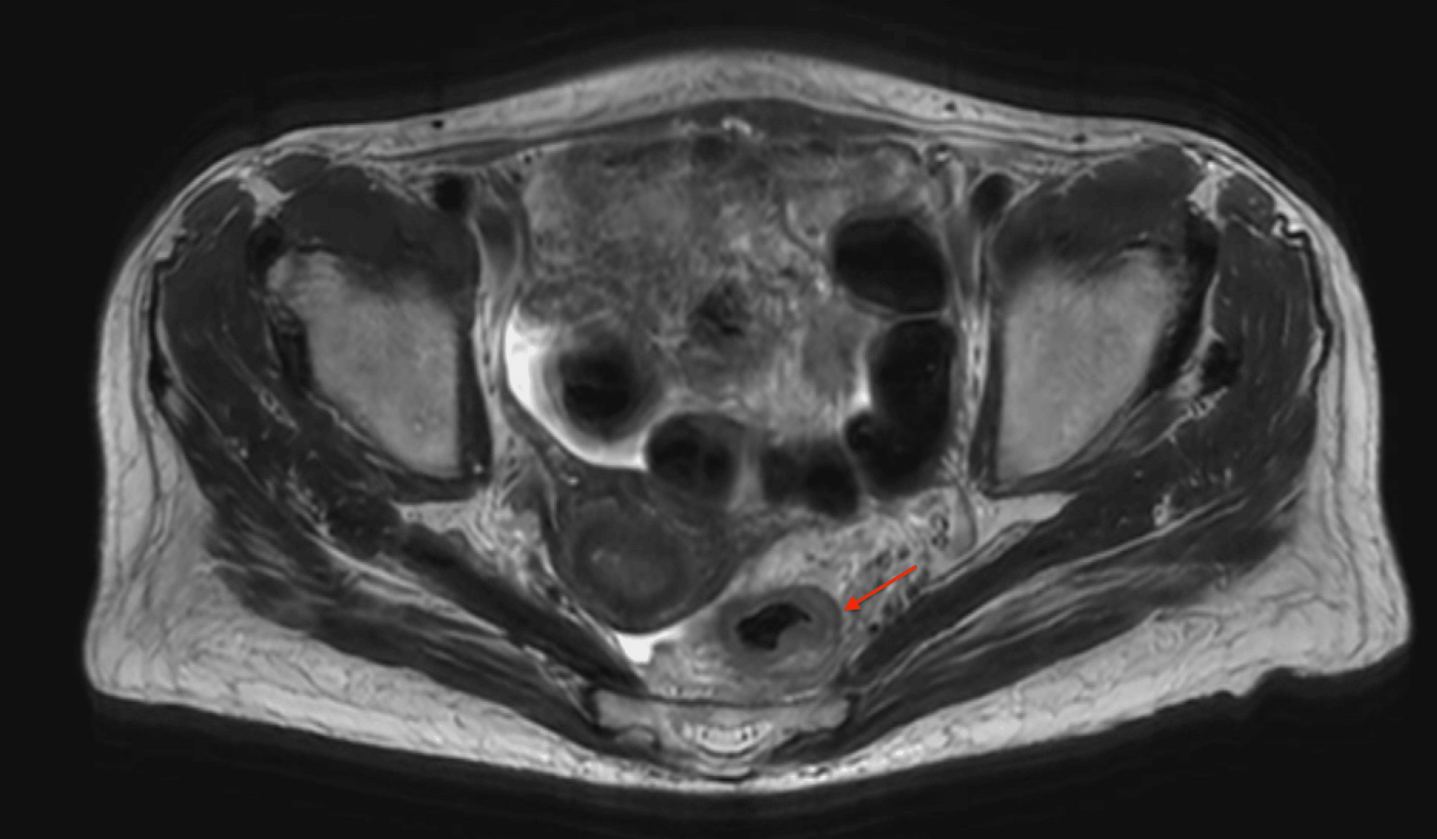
Axial T2-weighted pelvic MRI demonstrating asymmetric rectal wall thickening (arrow) at the site of the primary tumor, consistent with T3 disease These findings necessitated consideration of multimodal therapy and posed a clinical dilemma regarding the addition of pelvic radiation in a patient with prior radiation exposure.
